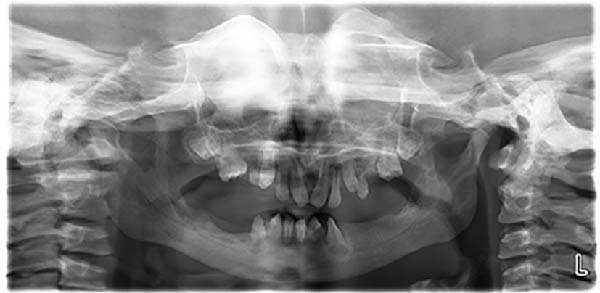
Orthopantomogram showing the initial condition.
